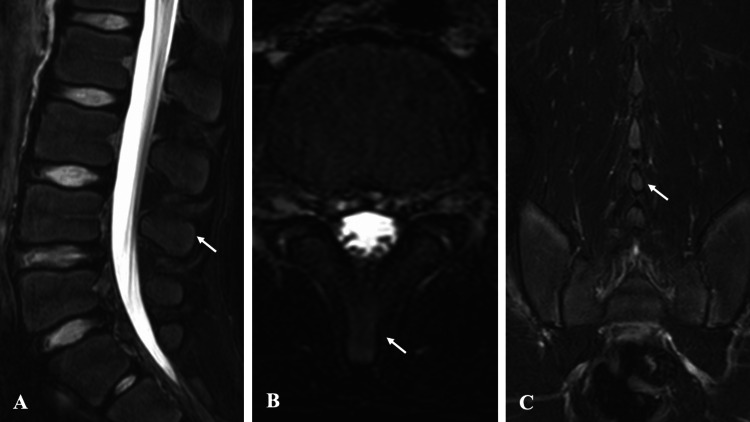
MRI findings in Case 2 three months after treatment. STIR sequence MRI shows resolution of high signal intensity at the L4 spinous process: (A) sagittal view, (B) axial view, and (C) coronal view. MRI, magnetic resonance imaging; STIR, short-tau inversion recovery
